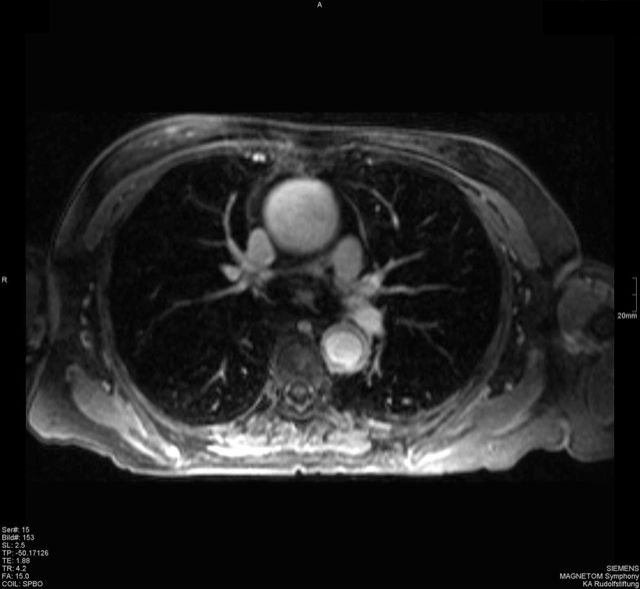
Thoracic CT from May 2004 - Thoracic CT from May 2004 showing the ectatic ascending aorta with a diffuse wall thickening.
